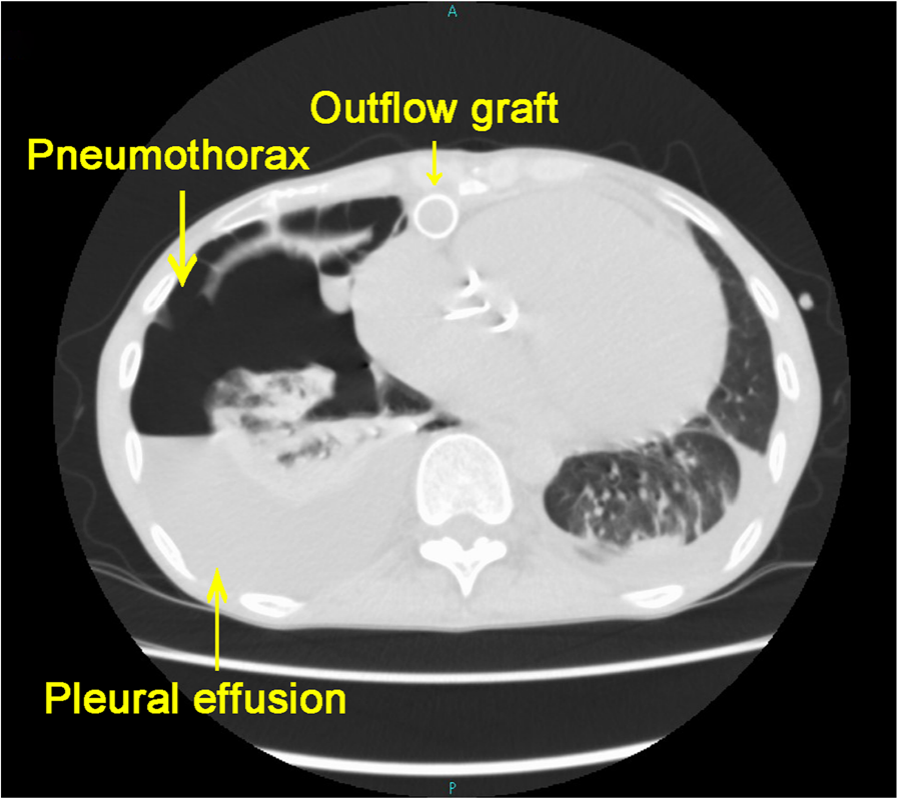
A computed tomography image of the chest in lung window. Computed tomography showing the right-side pneumothorax with moderately retained pleural effusion
